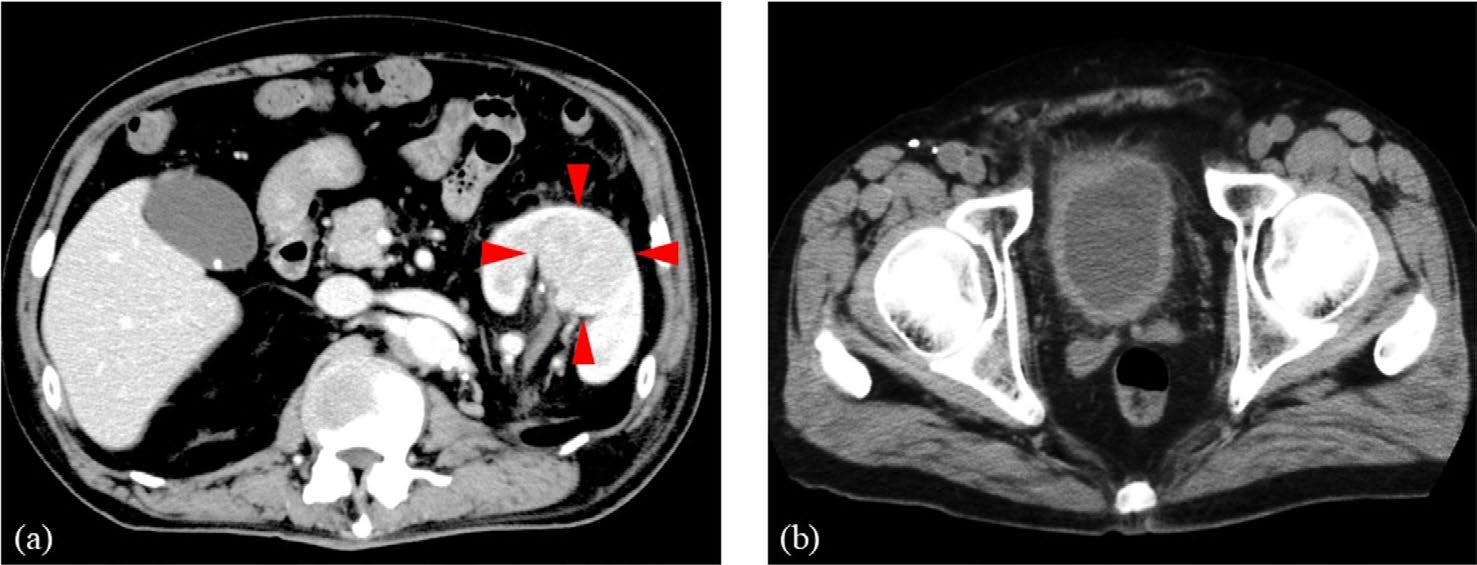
Contrast‐enhanced CT when the patient presented with persistent fever. (a) CT shows a 48 × 40 mm^2^ abscess in the left kidney. (b) CT shows no obvious bladder tumor.
